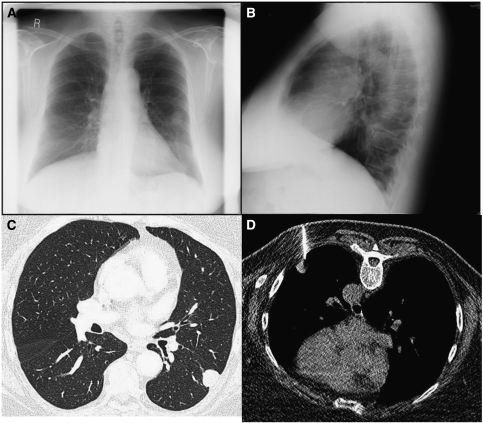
True positive. Chest X-ray reveals a ‘coin’ lesion in the left mid-zone (A), also demonstrated on the lateral radiograph (B). CT examination (C) on lung-window settings supports the diagnosis of a likely lung metastasis, later confirmed by CT-guided biopsy, performed with the patient lying prone (D).
